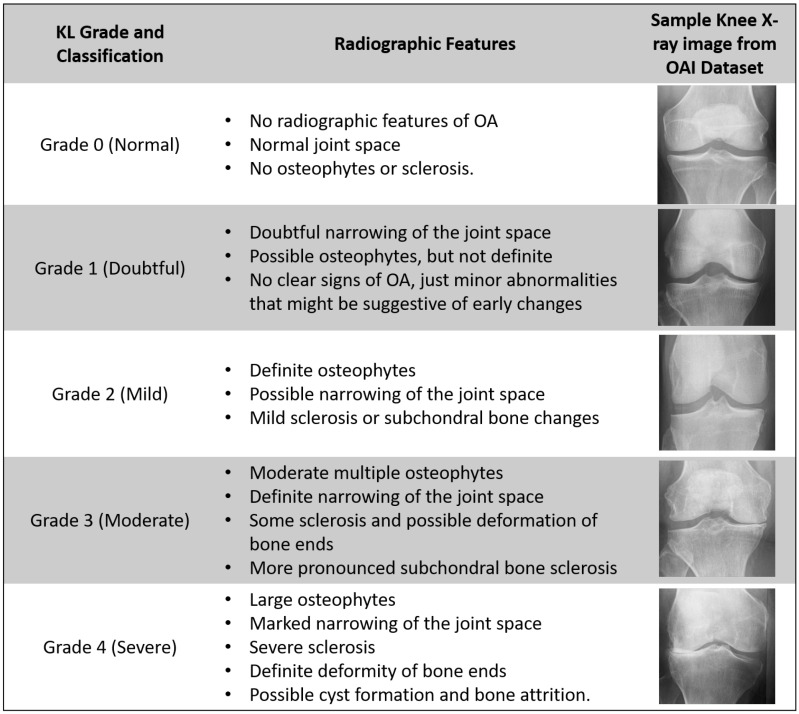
Kellgren–Lawrence grading scale for knee osteoarthritis with representative X-ray images.
